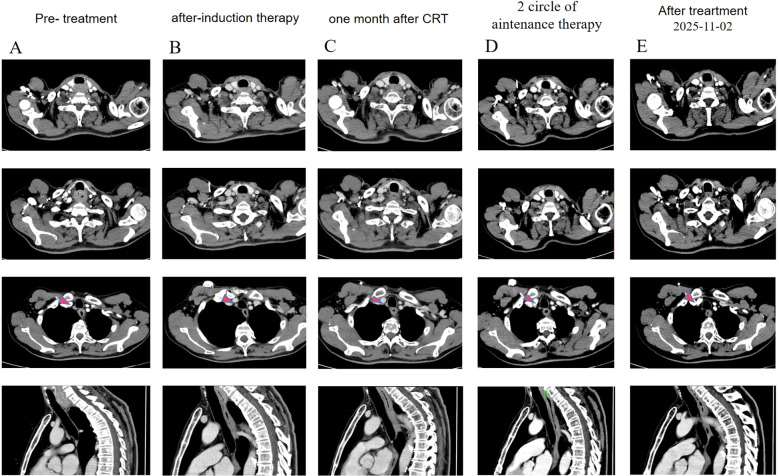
Images from pre- and post-treatment. (A) Pre-treatment baseline contrast-enhanced chest CT (axial and sagittal views). (B) Restaging after 2 cycles of induction therapy. (C) Follow-up 1 month after definitive concurrent chemoradiotherapy. (D) Restaging after 2 cycles of maintenance immunotherapy. (E) Recent follow-up after completion of therapy.
